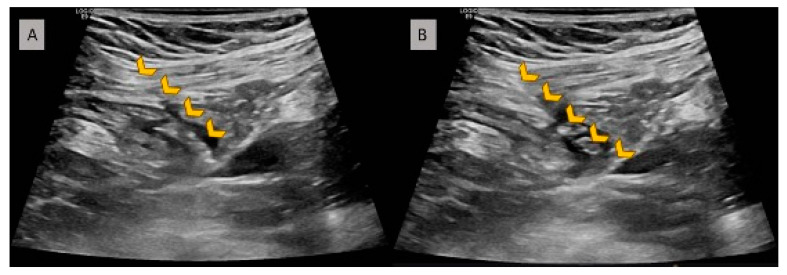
Images of ultrasound-guided axillary vein puncture (long-axis view—in-plane technique). The yellow markers indicate the needle. (A) Needle just before reaching the axillary vein. (B) The needle tip is inside the vessel lumen.
